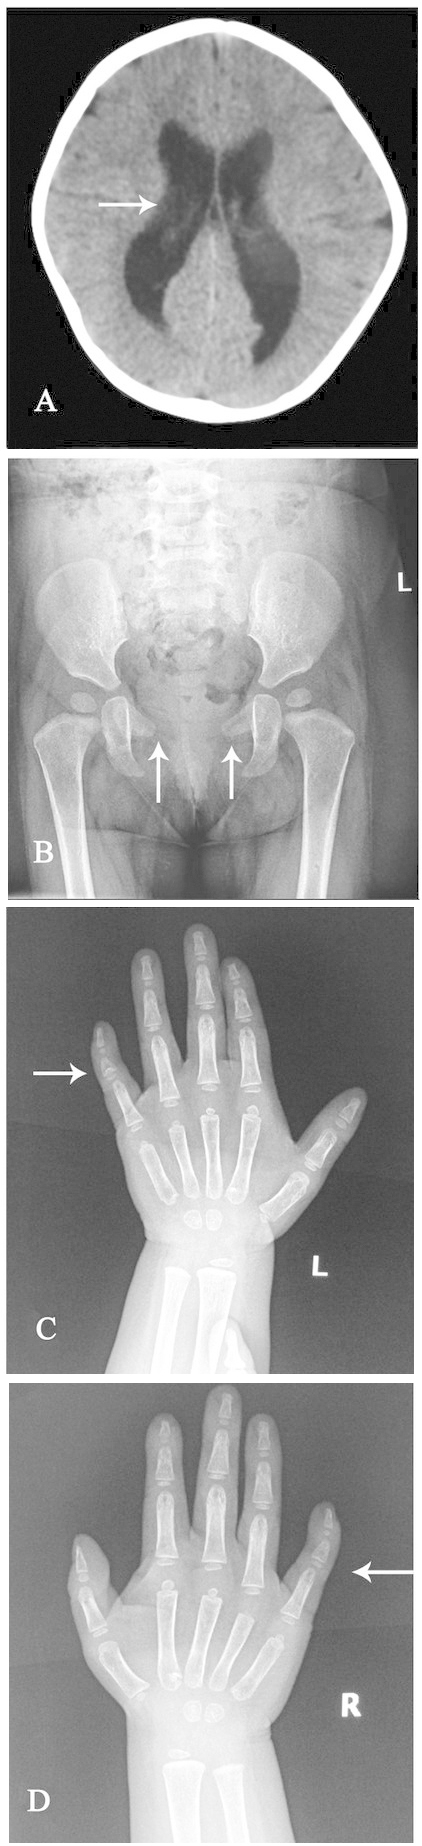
Patient analysis. (A) Cerebral computerized tomography showed enlargement of the lateral ventricles. (B) Roentgenogram of the hypoplastic pubic bones. Roentgenogram of the (C) left hand and (D) right hand, shows the left is smaller than the right and there is clinodactyly of both 5th fingers.
